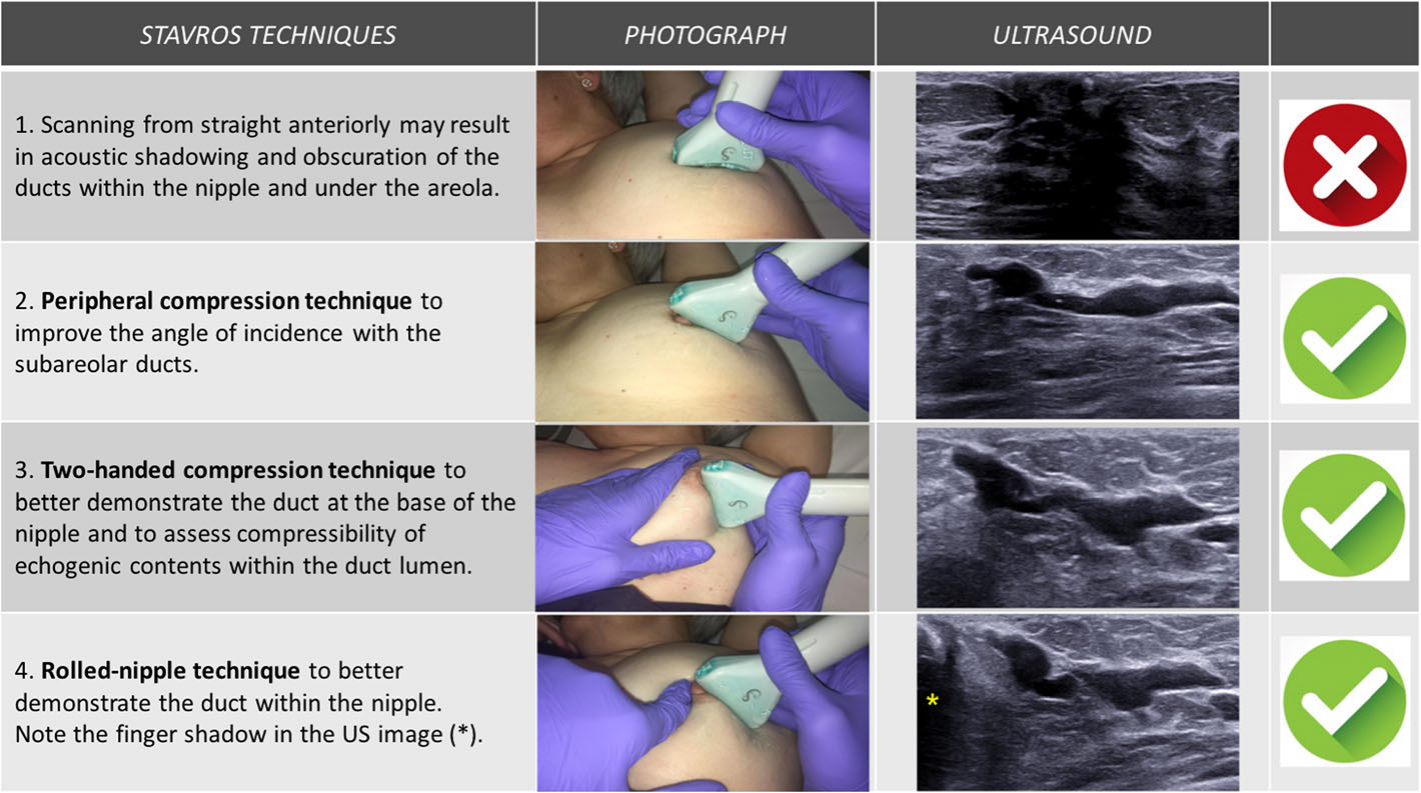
US techniques. Stavros ultrasound techniques to best demonstrate the subareolar and intranipple ducts
